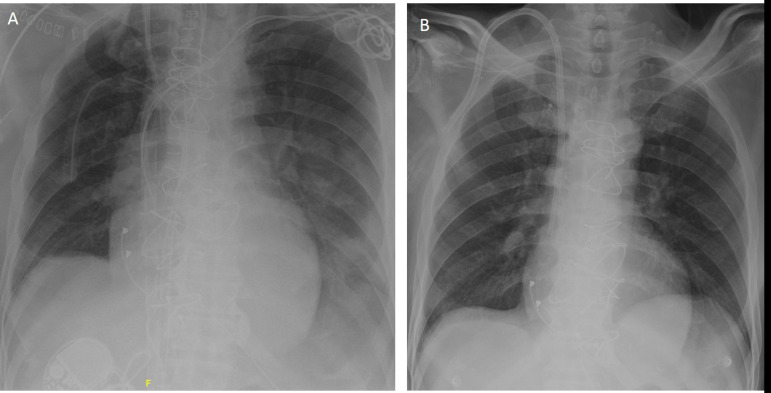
(A) Chest radiography after triple valve surgery, with remaining lead fragments and venous ECMO cannula in the right atrium. (B) Chest radiography after lead extraction and ECMO explantation.
